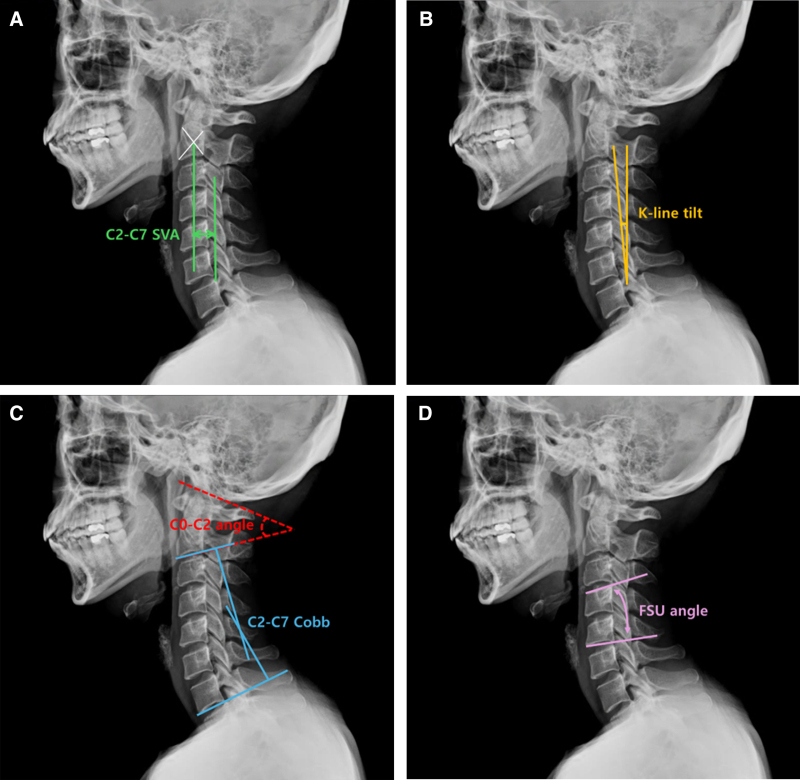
Radiographic parameters used to assess cervical sagittal alignment. (A) C2–C7 SVA, (B) K-line tilt, (C) C0–C2 angle (red line) and C2–C7 Cobb angle (blue line), (D) FSU angle. FSU = functional spinal unit, SVA = sagittal vertical axis.
